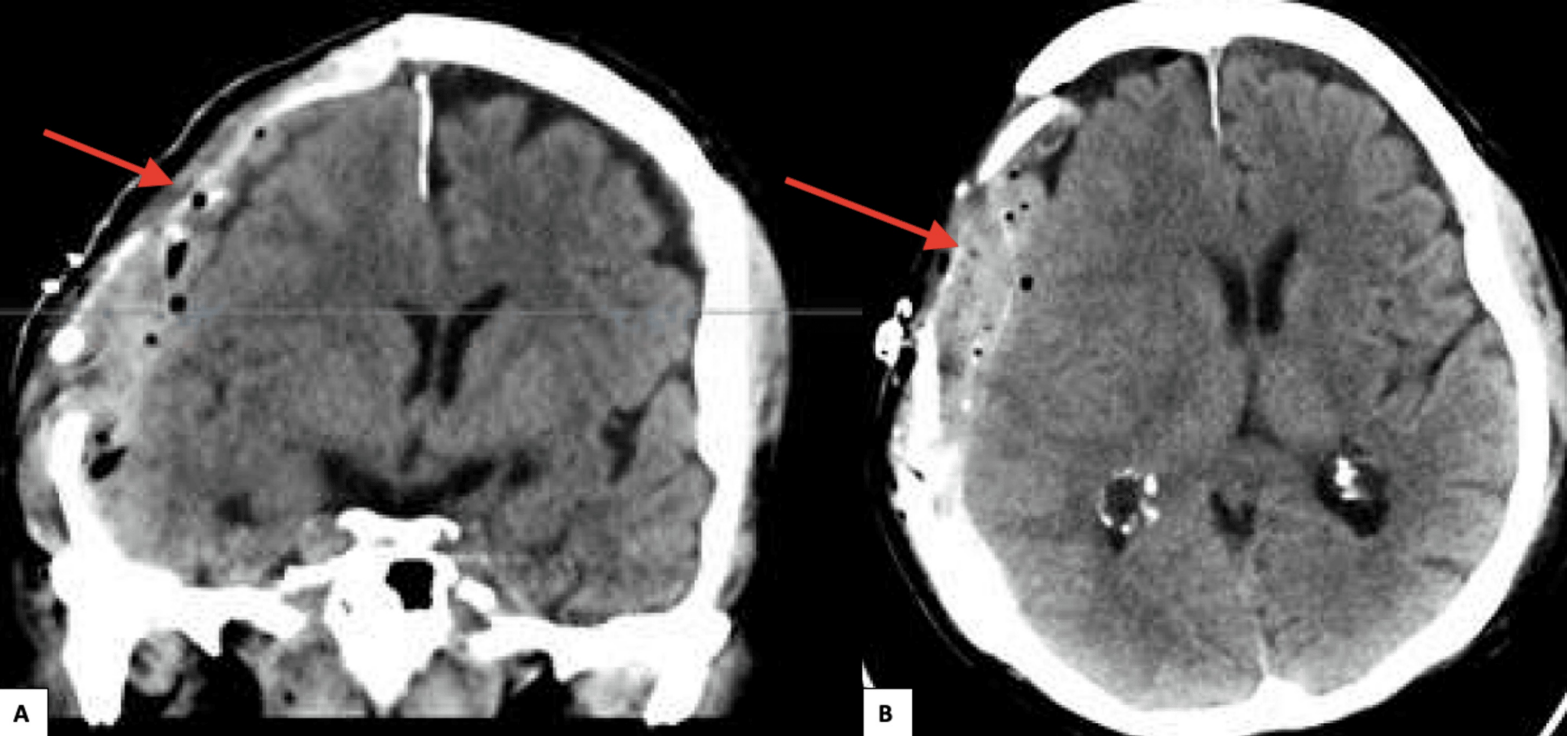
CT scan showing narrow subdural hematoma in the right frontotemporoparietal region (A: coronal; B: axial). The blue line in the coronal section represents the axial cut section accordingly.
